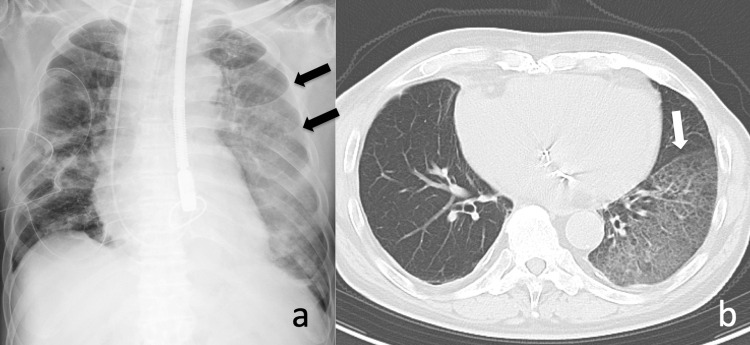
Postoperative images. a) Radiograph of the chest shows ground-glass opacities in the left lung on postoperative day 0. b) Postoperative plain computed tomography of the chest shows ground-glass opacities in the entire left lower lobe, interpreted as alveolar hemorrhage.
